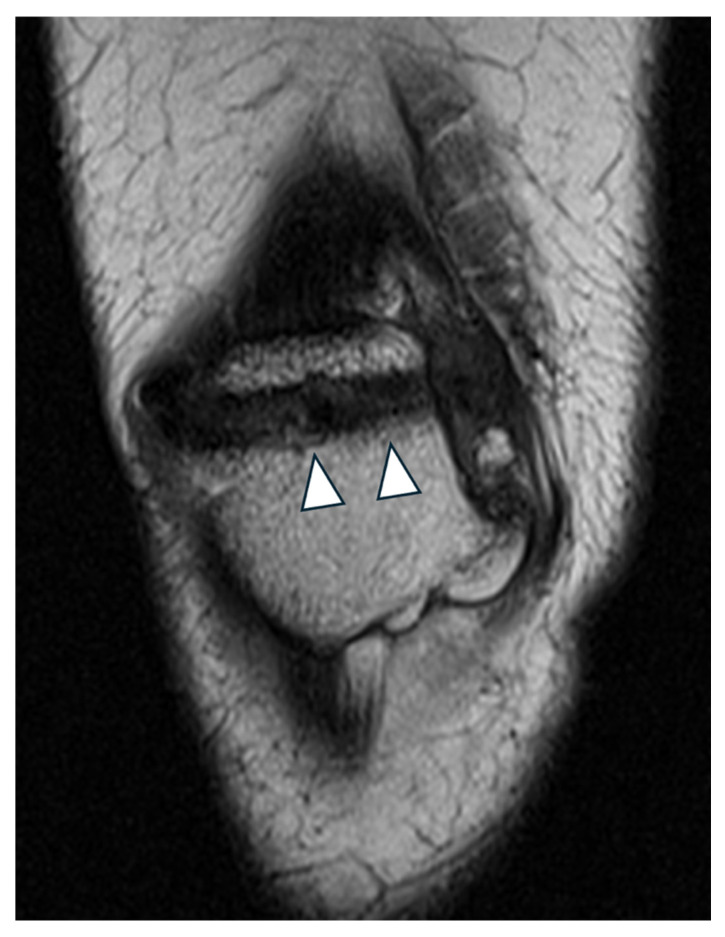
Coronal MRI of the patella in a patient with previous MPFL reconstruction. A semitendinosus graft was passed transversely through the patella (arrowheads) and fixed with an adjustable loop button (not shown).
